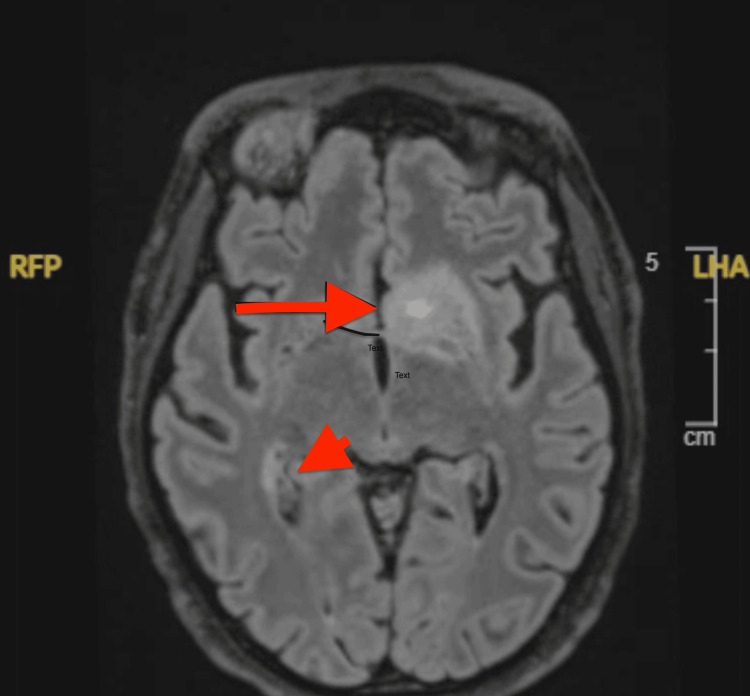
MRI of the brain showing infarction in case 2
